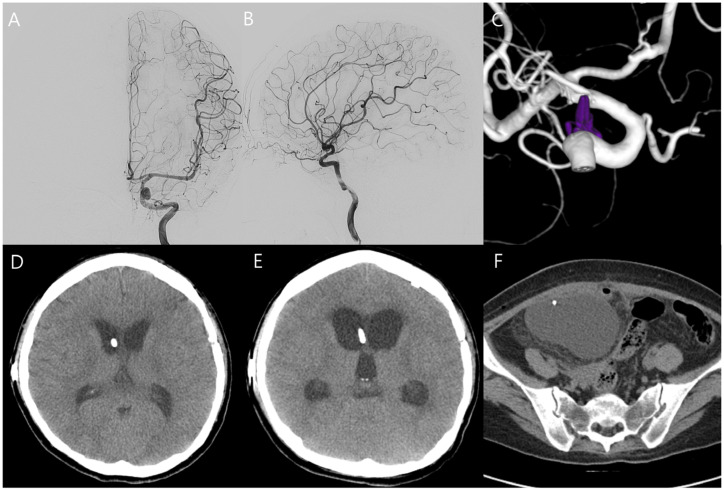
(A,B) Follow-up angiography after delivery reveals resolution of vasospasm and (C) 3D rotational angography demonstrates complete occlusion of the aneurysm. (D) Follow-up brain and abdomen CT at 11 days after ventriculo-peritoneal shunt, reveal recurrent hydrocephalus due to shunt failure with pseudocyst formation in abdominal cavity (E,F).
